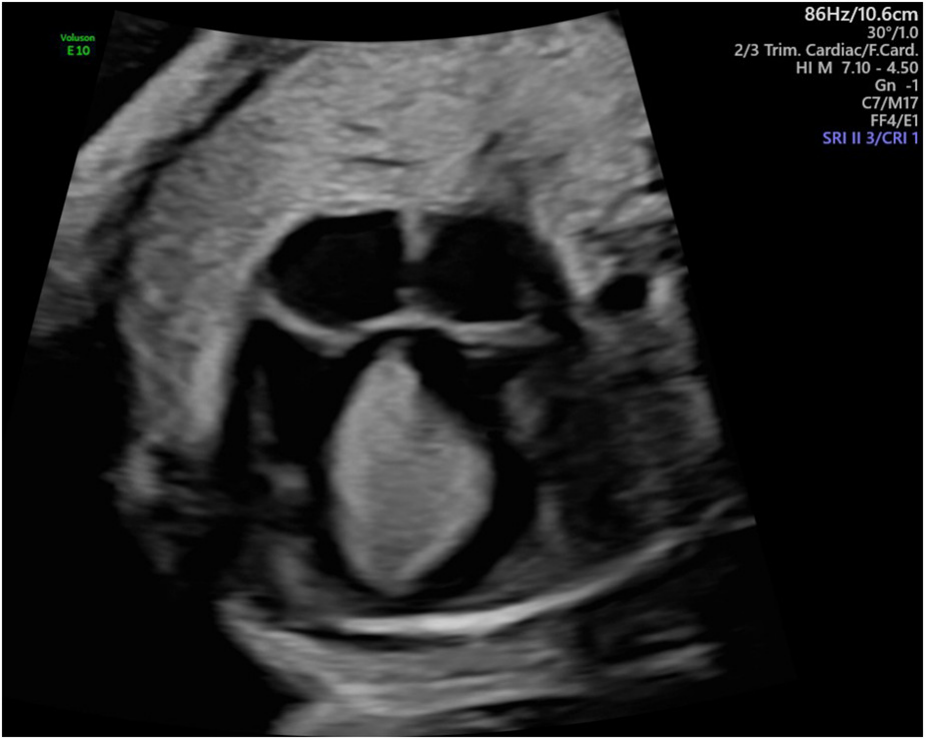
Fetal echocardiography at the time of cessation of transplacental sirolimus therapy: four-chamber view showing the septal tumor mass, now slightly reduced in size.
